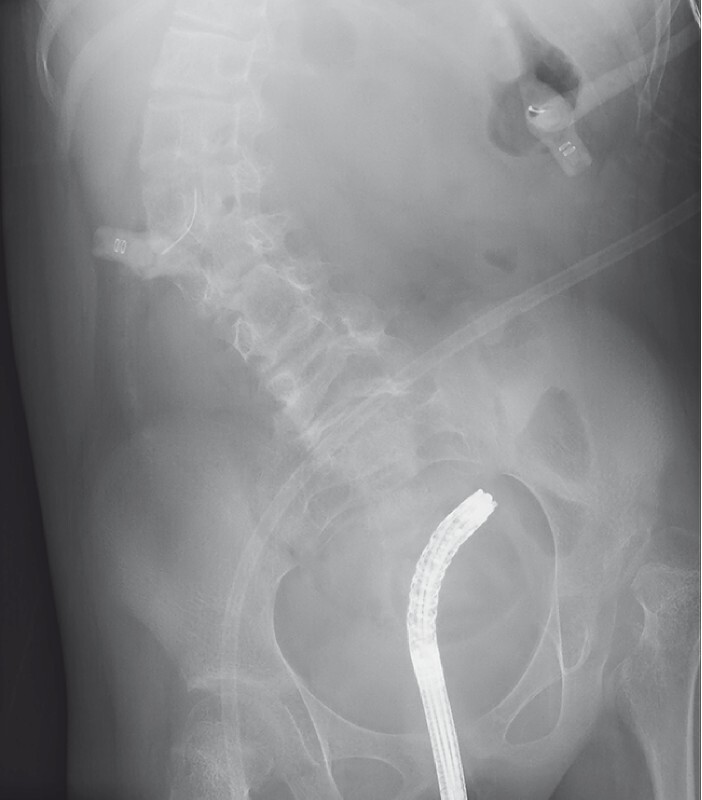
X-ray fluoroscopy showed that the dilated colon had disappeared.
